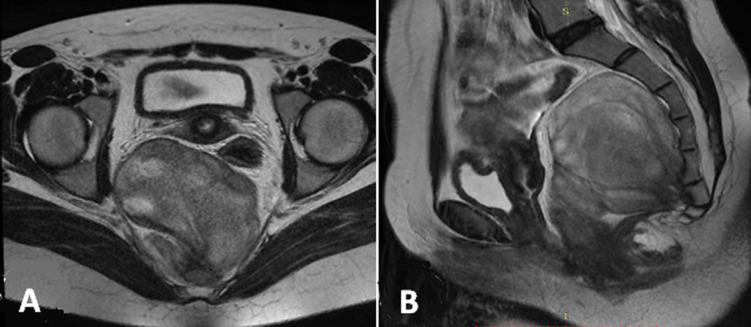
pelvic MRI T2-weighted images: A) axial; B) sagittal
